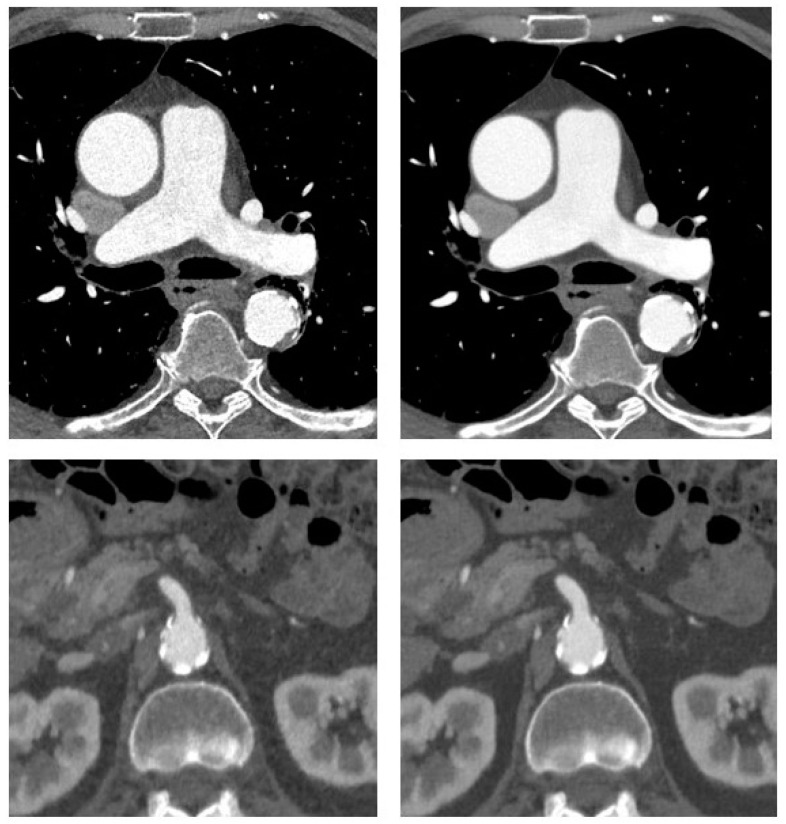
CT angiography of the aorta in a 72-year-old man with coronary artery disease and suspected aneurysm of the ascending aorta. Transverse sections at the level of the ascending aorta and superior mesenteric artery origin are shown with adaptive statistical iterative reconstruction V (ASIR-V 60%, left) and deep-learning-based reconstruction (DLIR-H, right). There is mild aneurysmatic dilatation of the ascending aorta with a maximum diameter of 4.3 cm. Some atherosclerotic plaque along the descending aorta is also noted.
